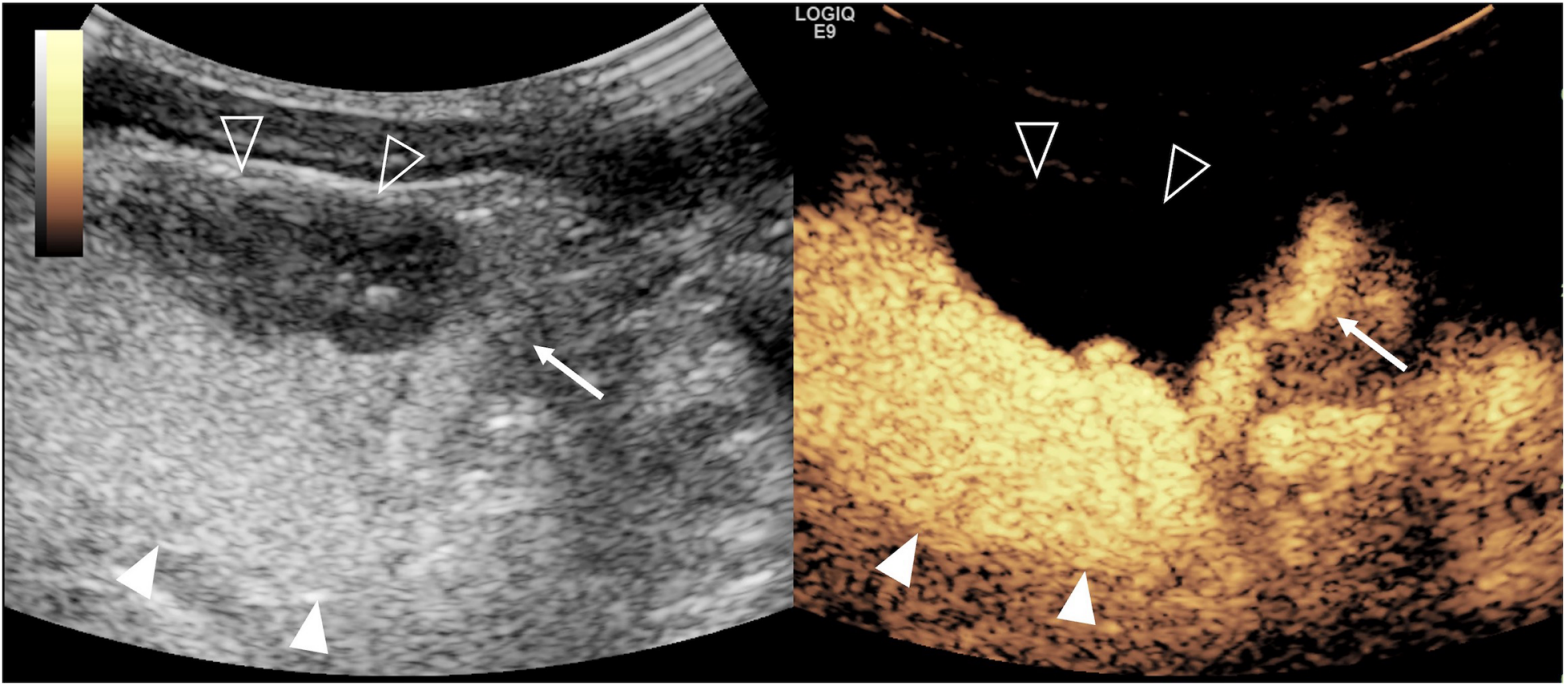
Duplex pelvis complicated with vesicoureteral reflux on contrast-enhanced voiding urosonography (ceVUS) of the dual-screen mode of the left kidney in a 14-day-old girl. Sagittal gray-scale ultrasound (left) and ceVUS (right) images show hydronephrosis of the upper moiety (solid arrowhead) of the duplex kidney and microbubbles refluxing and filling the upper moiety pelvicalyceal (solid arrowheads) with marked dilation and papillary impressions invisible and tortuous dilated ureter (arrow), indicating grade V reflux. There is no dilation and reflux of the lower moiety pelvicalyceal system (open arrowheads).
